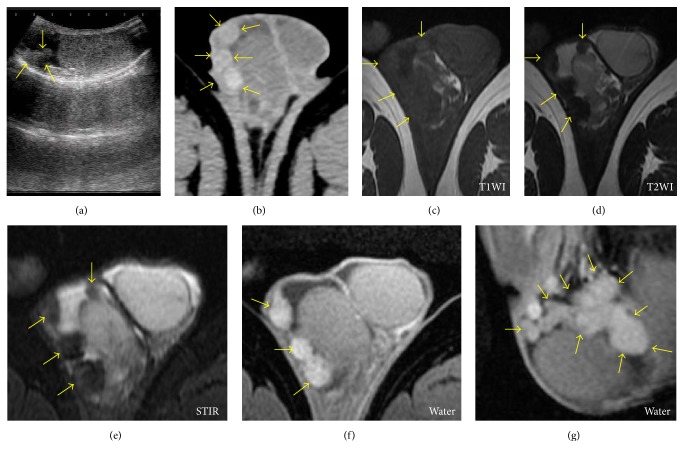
Imaging of the fibrous pseudotumor in the scrotum*. Scrotal ultrasound revealed a normal testicle and multiple 3 to 7 mm hyperechoic lesions adjacent to the right testis ((a), arrows). Contrast-enhanced computed tomography (CT) showed high-density paratesticular tumors ((b), arrows). Magnetic resonance imaging (MRI) showed iso- and low-intensity paratesticular tumors in T1- ((c), arrows) and T2-weighted MR images ((d), arrows), respectively. Short TI inversion recovery (STIR) MRI showed low-intensity paratesticular tumors ((e), arrows). Water MRI showed high-intensity tumors ((f) and (g), arrows). T1WI: T1-weighted image; T2WI: T2-weighted image.
